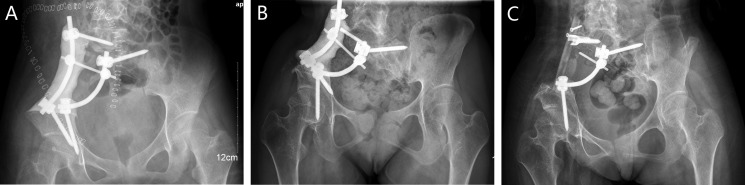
A 16-year-old female (case 4) with diagnosis of pelvis osteosarcoma affecting zone I + IV (A) AP radiograph at one week post-operatively. (B) AP radiograph at 61.9 months post-operatively, showing failure of the implant, and the implant was stabbed to the skin when she stooped, (C) AP radiograph after the construction was revised and augmented with an autologous fibular graft 80 months post-operatively.
